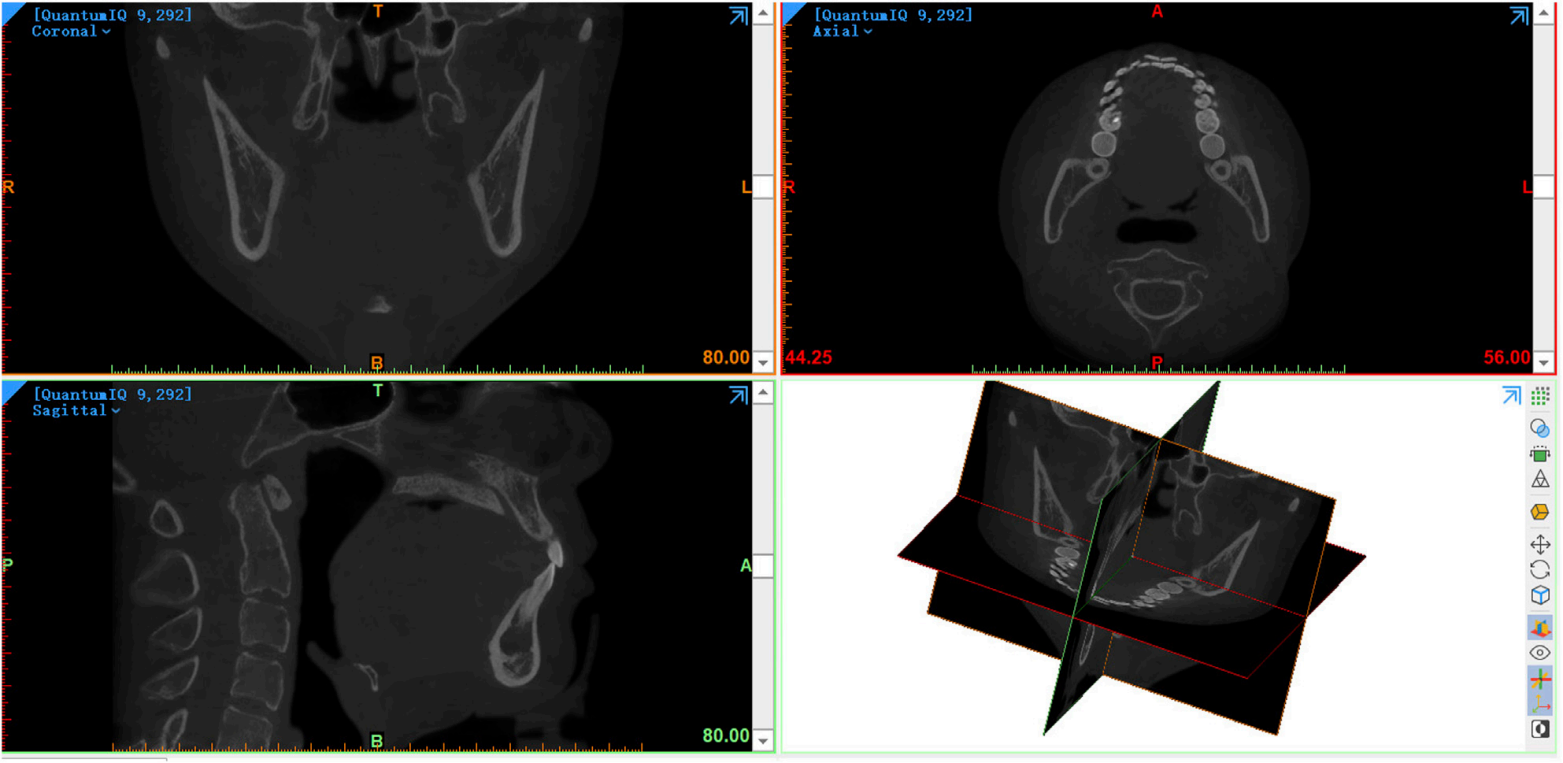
The CBCT data in DICOM format was imported into mimics21 software.
